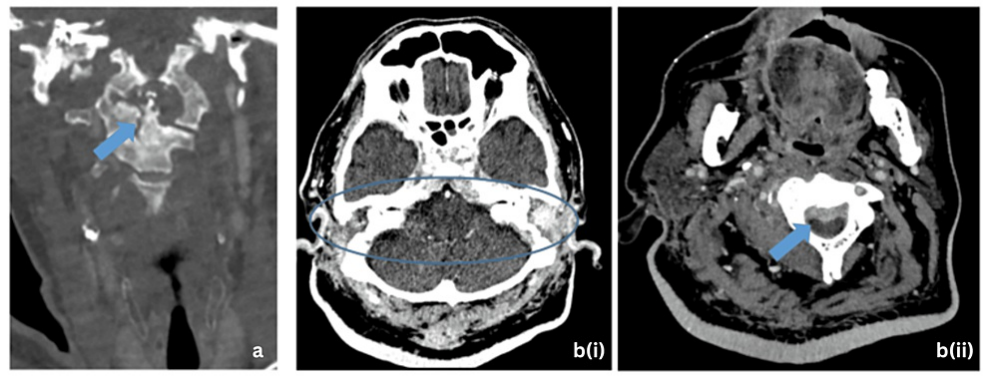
(a) Coronal contrast-enhanced CT image in the bone window shows further progression of the cortical erosion of the basisphenoid, clivus, dens of C2 vertebra (arrow), and the posterior aspect of C3 and C4 vertebra bodies. (b)(i) Axial contrast-enhanced CT image in soft tissue window shows smooth diffuse mucosal thickening and enhancement of the cartilaginous and osseous segments of the bilateral external auditory canal (circle) (b)(ii) with prevertebral soft tissue enhancement causing mild spinal canal narrowing anteriorly, worst at the C2 vertebral level (arrow). These findings were interpreted as progressive inflammatory changes.
